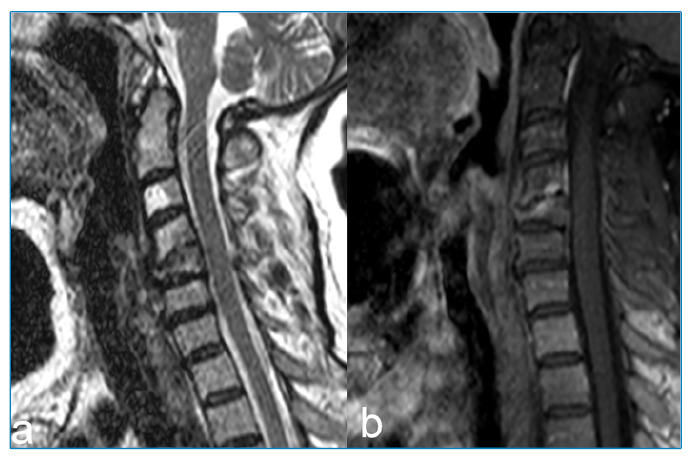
Post-treatment cervical magnetic resonance images. Almost complete regression can be seen in abscess formations in the prevertebral and anterior epidural distance on sagittal T2 AG (a) and fat-suppressed contrast-enhanced T1 AG (b) images
